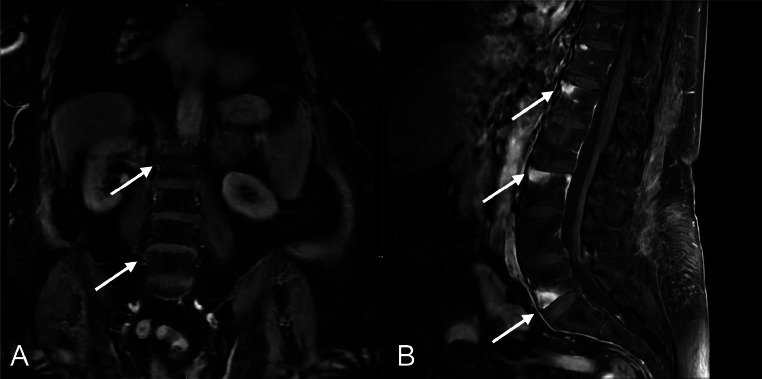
(A) MRI Abdomen 2023 T1 FS contrast enhanced sequence. L2-L5 vertebral bodies (white arrows) are visualized without end plate or soft tissue enhancement. No sagittal images available. (B) Compared to MRI L spine 2024 T1 fat saturated contrast enhanced sequence which shows marrow edema and vertebral endplate enhancement is seen at L1, L3, and L4-L5 (white arrows).
